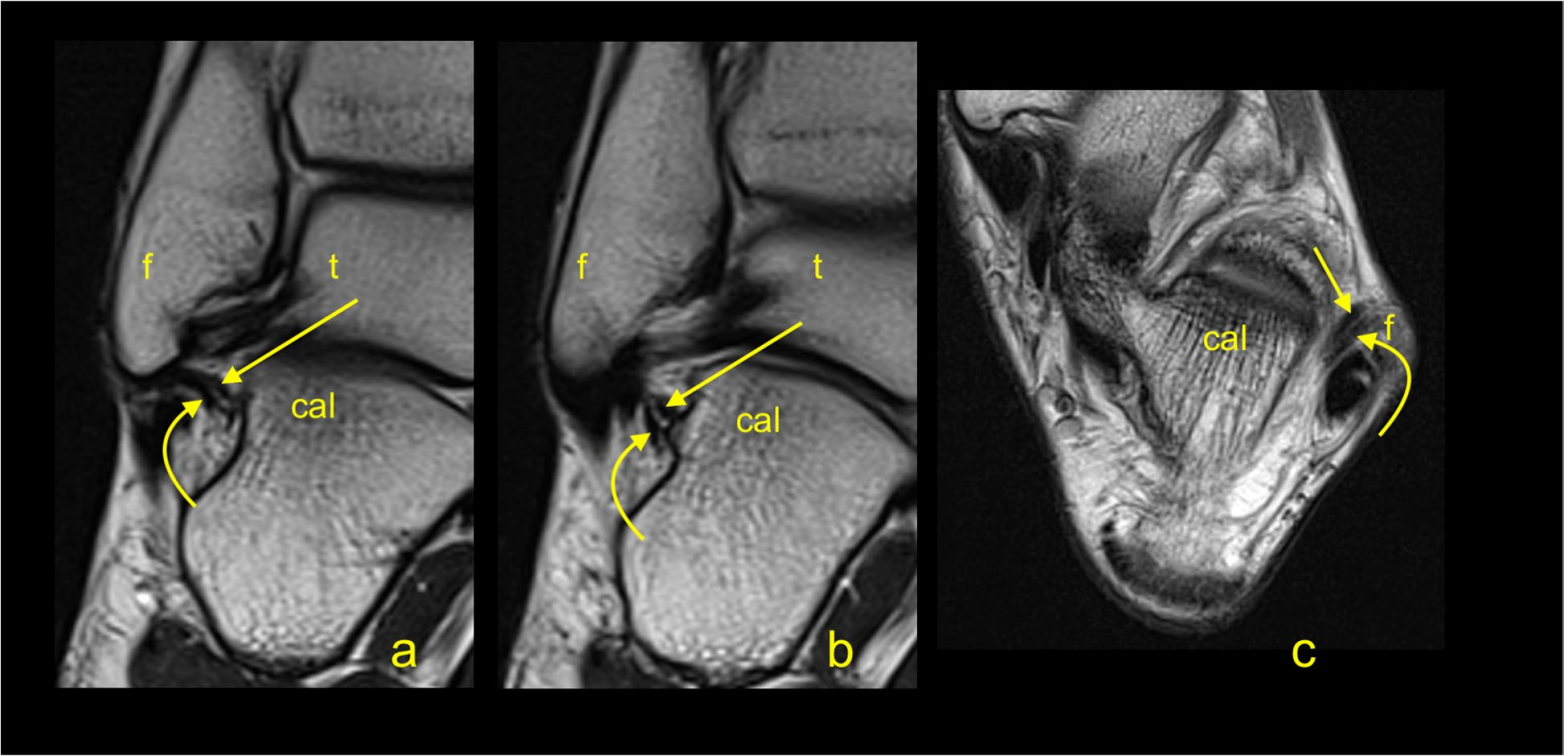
The double fascicular calcaneofibular ligament. The medial (arrow) and lateral fascicle (curved arrow) are visible. A and b T2-weighted coronal section; c T2-weighted axial section. Cal, calcaneus; f, fibula; t, talus
